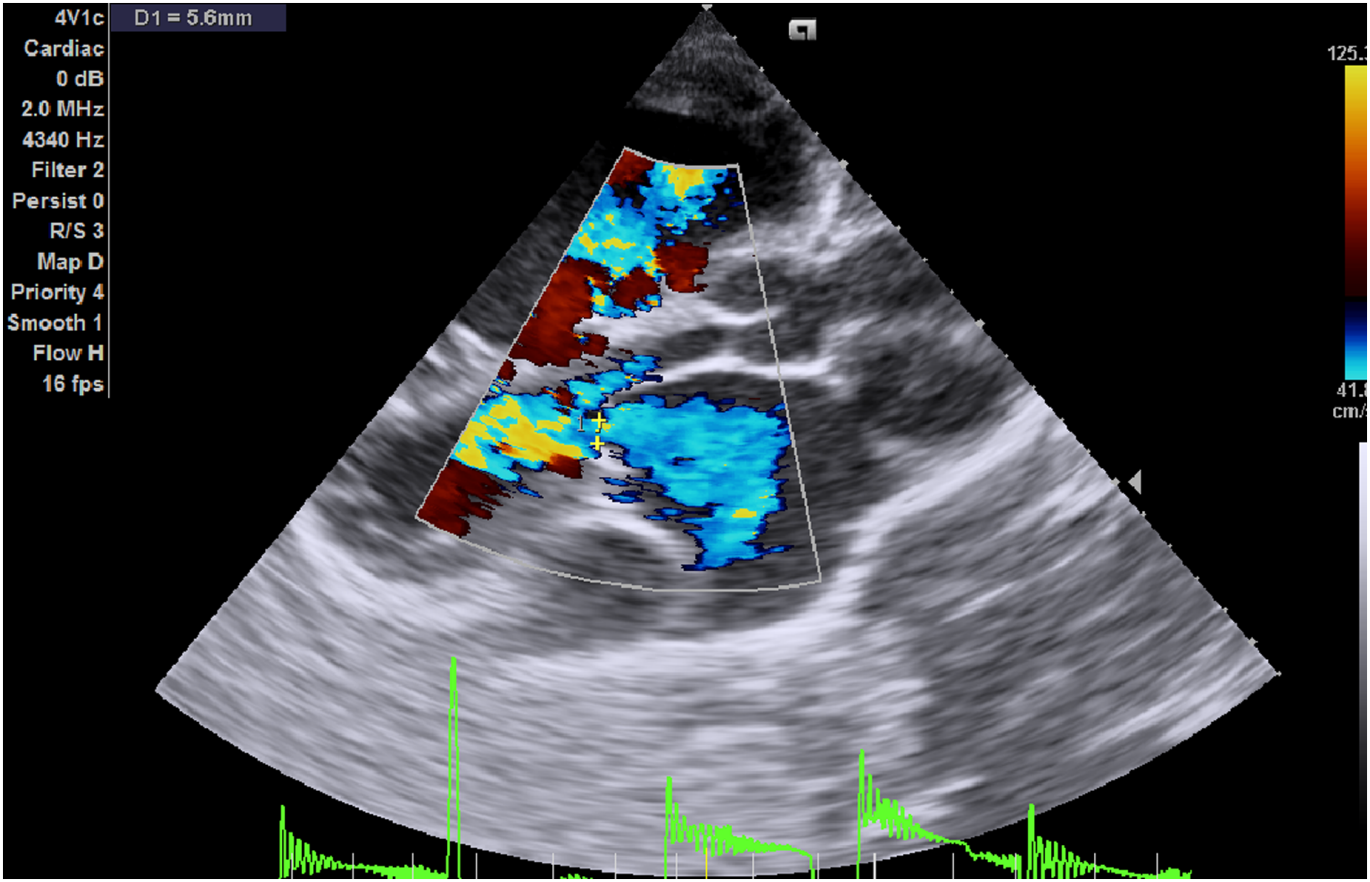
Epical 4 chamber color echocardiograph showing mitral regurgitation with vena contracta of 0.56 cm.
